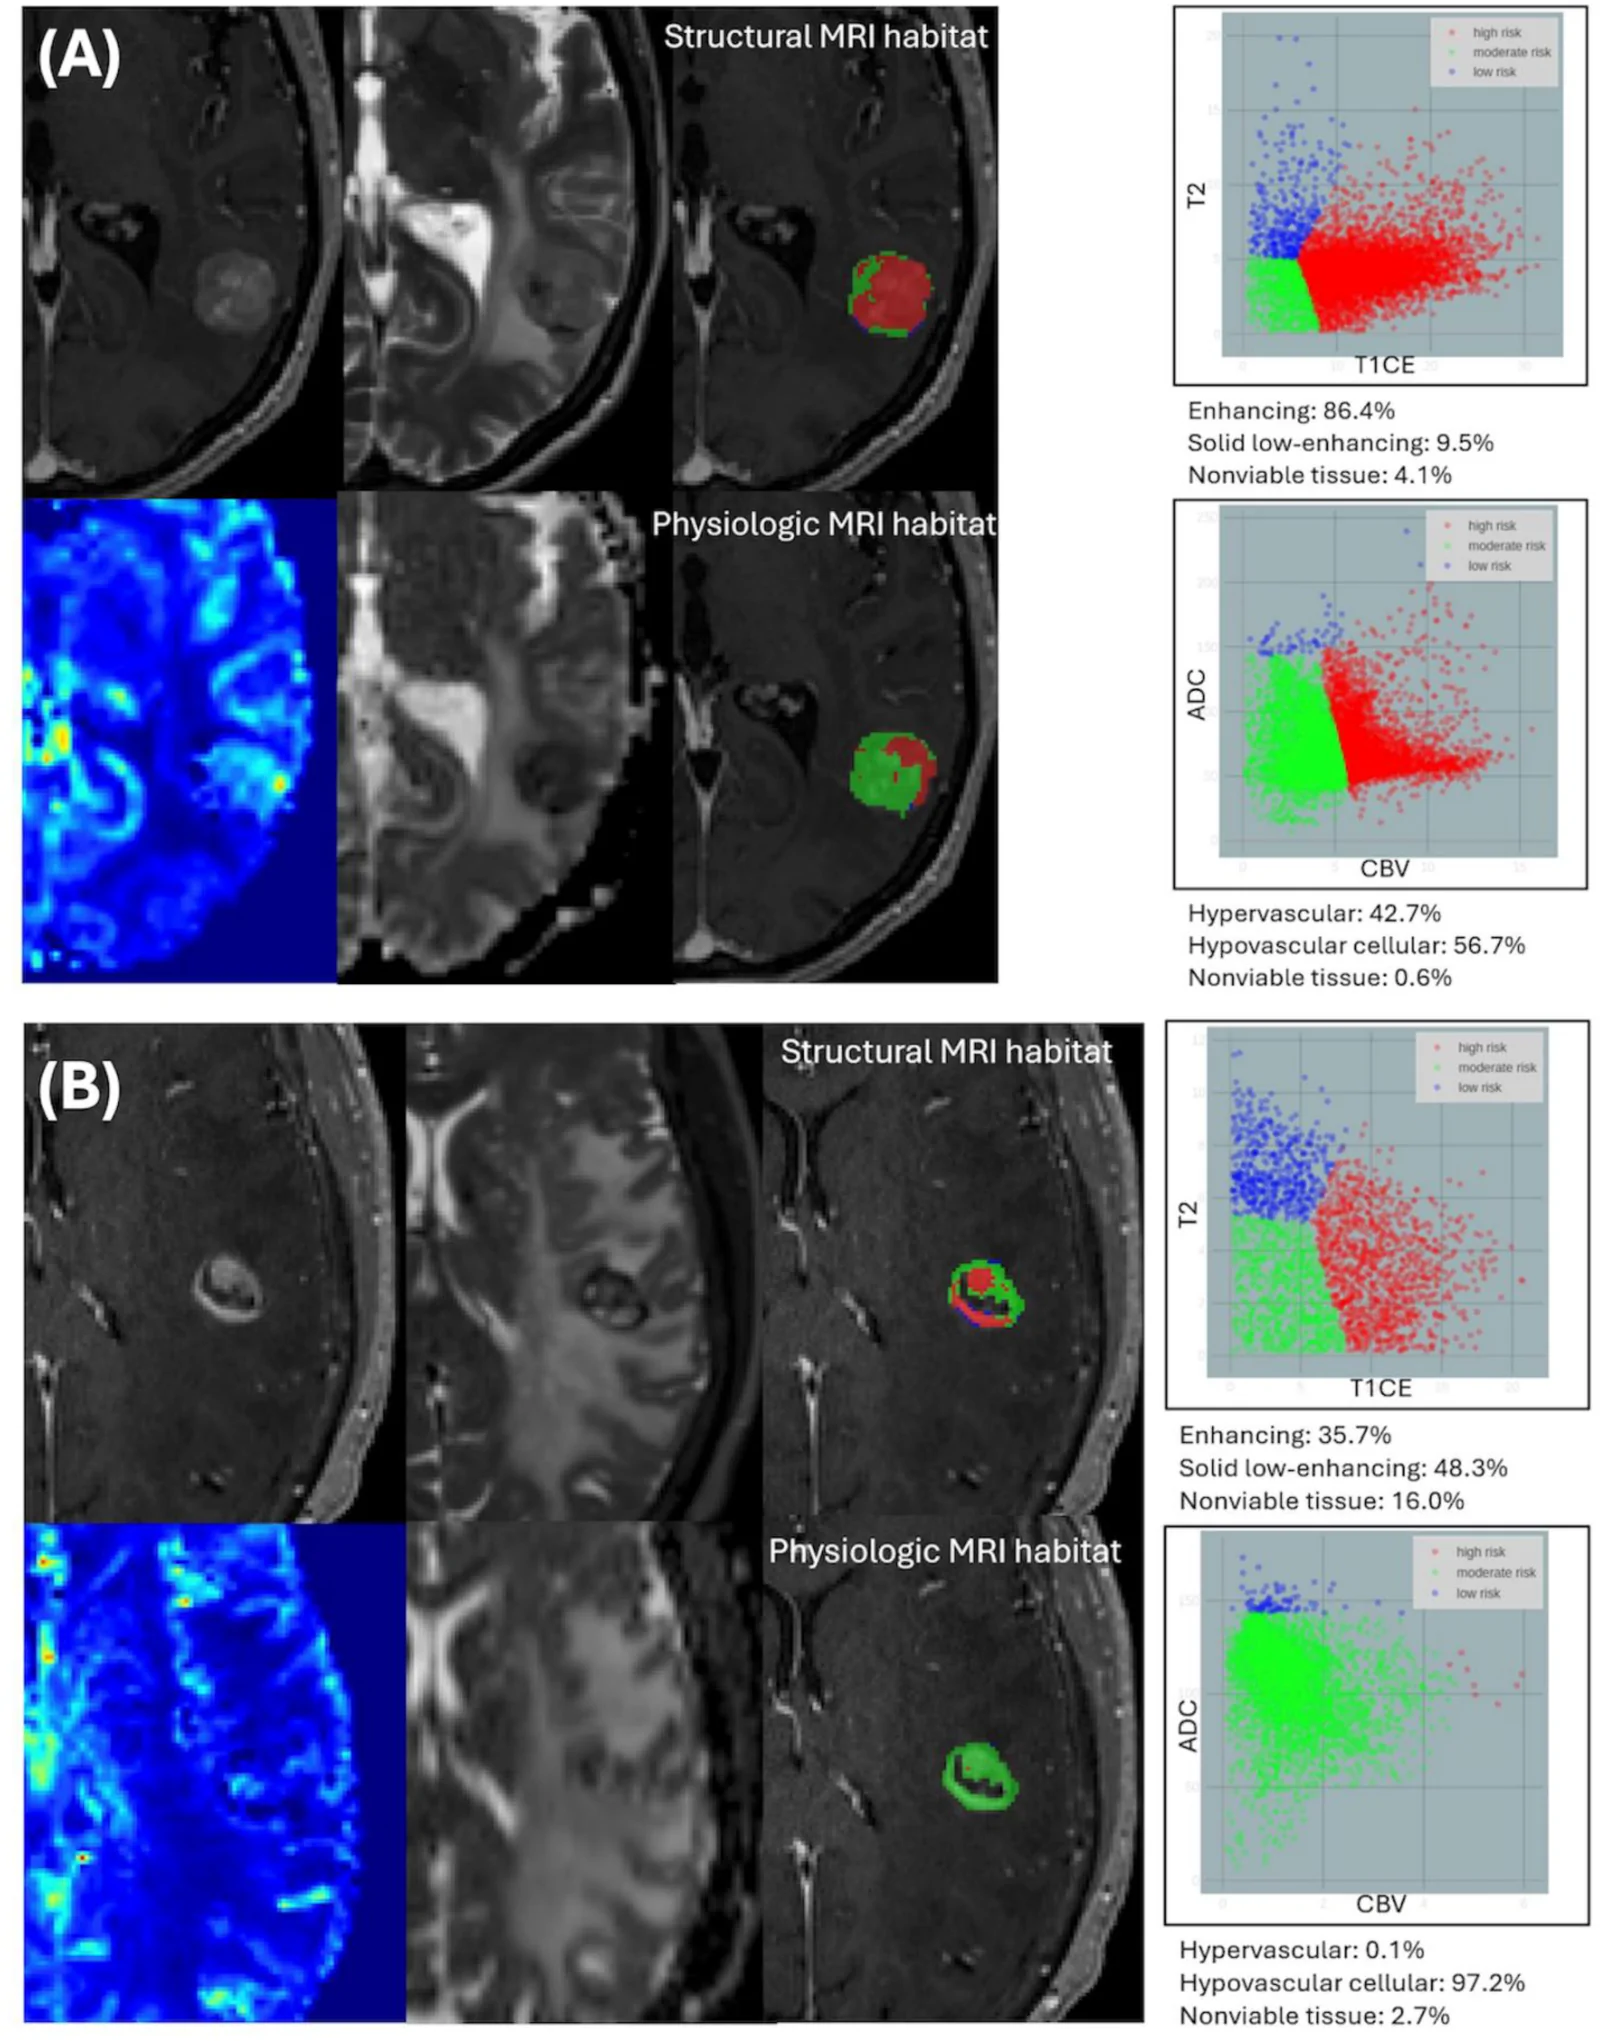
Comparison of structural MRI and physiologic MRI-based tumor habitat analysis in patients with tumor recurrence. (A) A 58-year-old female with breast cancer treated with SRS for the left temporal lobe lesion. On structural MRI, nonviable tissue habitat was scanty (4.1%) and enhancing habitat was predominant (86.4%). On physiologic MRI, prominent hypervascular habitat (42.7%) and scanty amount of nonviable tissue habitat (0.6%) is noted. (B) A 71-year-old female with melanoma treated with SRS for left temporal lobe lesion. On nonviable tissue was less than 16.5% on structural MRI (16.0%) and 10.1% on physiologic MRI (2.7%), respectively
